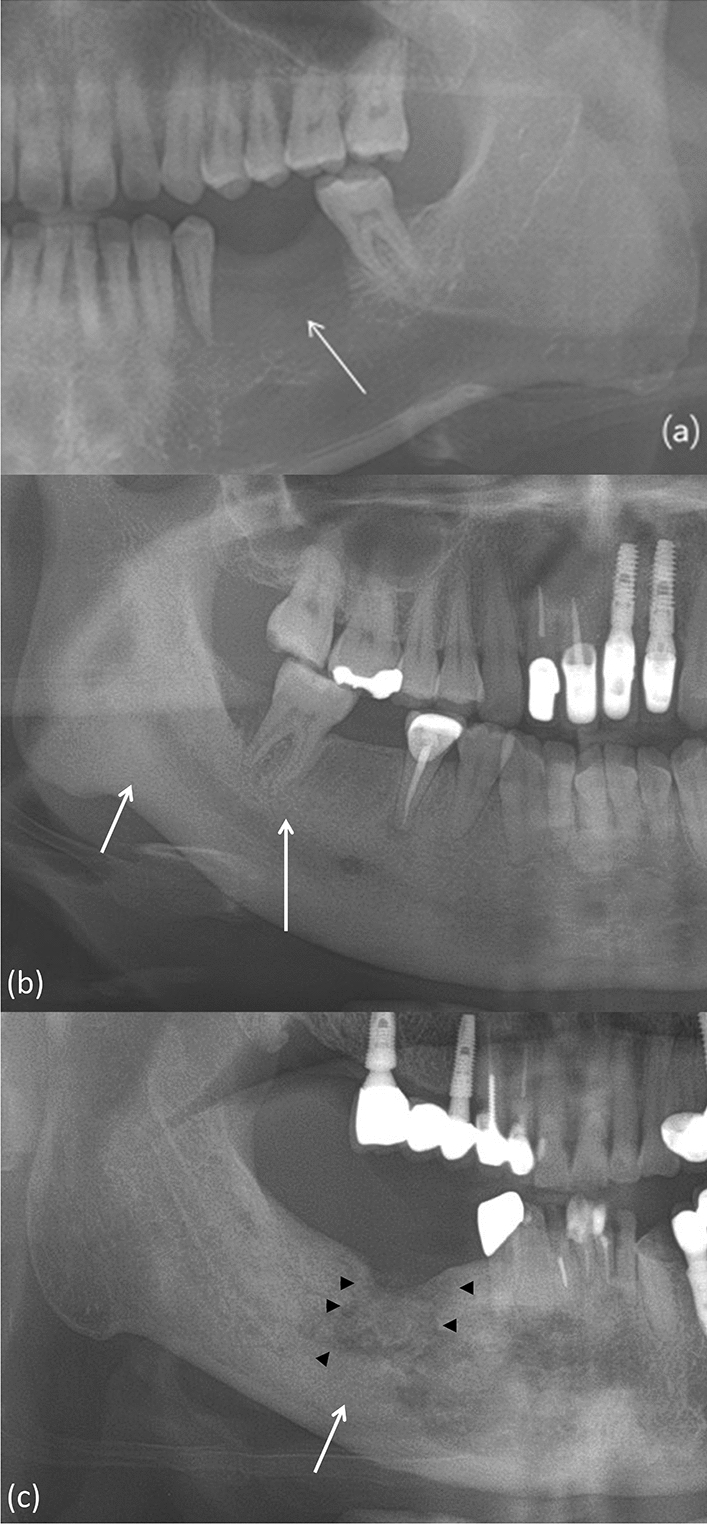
In panoramic radiographs, bone change patterns can be classified as (a) osteolytic bone change, as shown by the ill-defined radiolucency (white arrow) in the left premolar and molar region; (b) sclerotic bone changes, as indicated by the ill-defined diffuse radiopacity (white arrow) in the bone marrow; and (c) mixed osteolytic (black arrowheads) and sclerotic (white arrow) bone changes.
